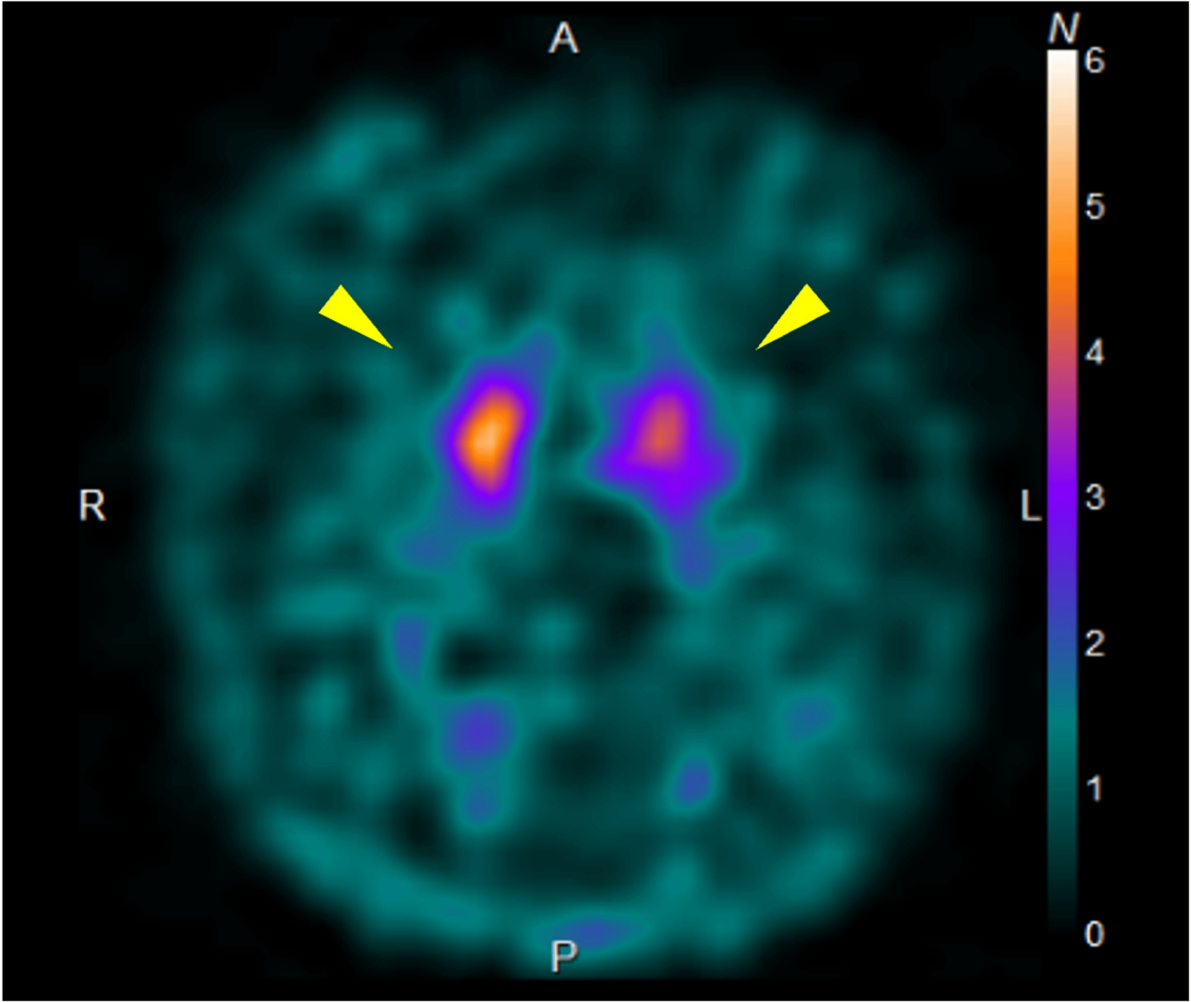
DAT-SPECT image showing reduced uptake. Yellow arrowhead indicates reduced uptake which is shown in both basal ganglia, predominantly on the left. The specific binding ratio (SBR) is 7.59 on the right and 6.09 on the left. DAT-SPECT: dopamine transporter single-photon emission computed tomography.
